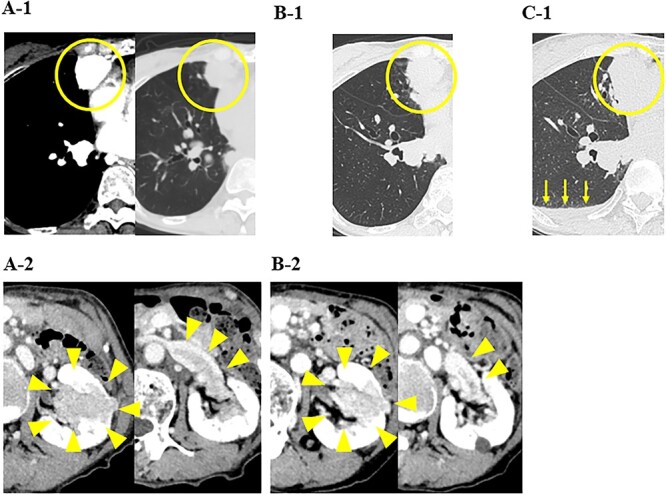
CT images of the chest and abdomen; (A) at the start of the administration of nivolumab and ipilimumab, CT showed multiple masses in both lung fields and a mass in the left kidney with a tumor plug in the left renal vein, and (B) 5 months after the administration of nivolumab and ipilimumab, CT showed increases in the sizes of the pulmonary tumors and a decrease in the size of the renal tumor, and (C) 6 months after the administration of nivolumab and ipilimumab, CT showed increases in the sizes of the pulmonary tumors and pleural effusion (yellow arrows).
